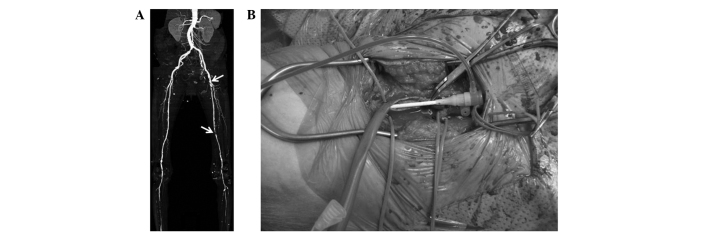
Femoral endarterectomy and endovascular treatment of a lesion in the SFA. (A) Preoperative CTA showing tight stenosis on the left CFA and SFA. (B) Image of the inserted introducer sheath for endovascular treatment of a lesion in the SFA. CTA, computed tomography-angiography; SFA, superficical femoral artery; CFA, common femerol artery.
